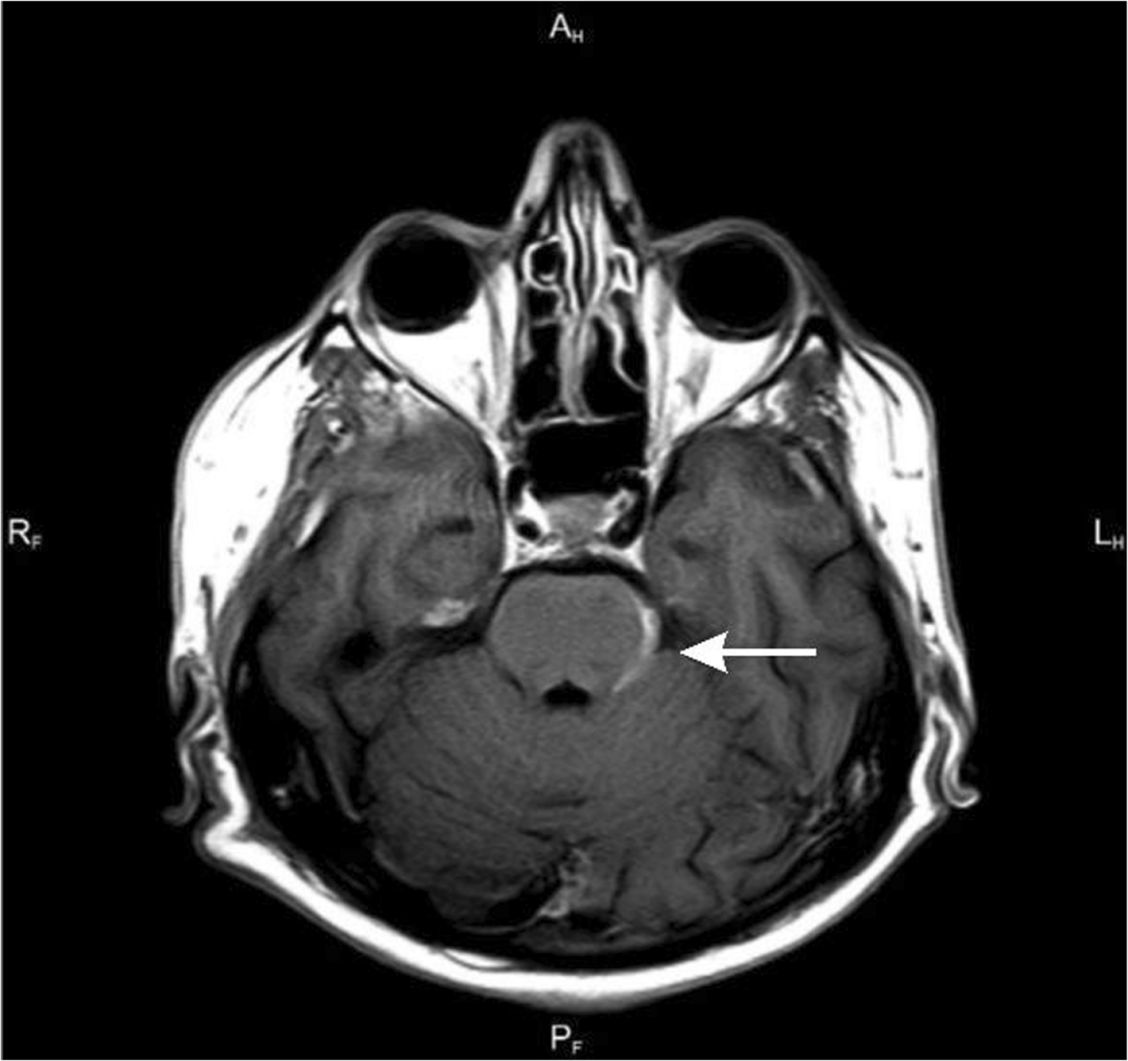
Axial T1-weighted scan after contrast enhancement - contrast enhancement of the left lateral surface of the pons
